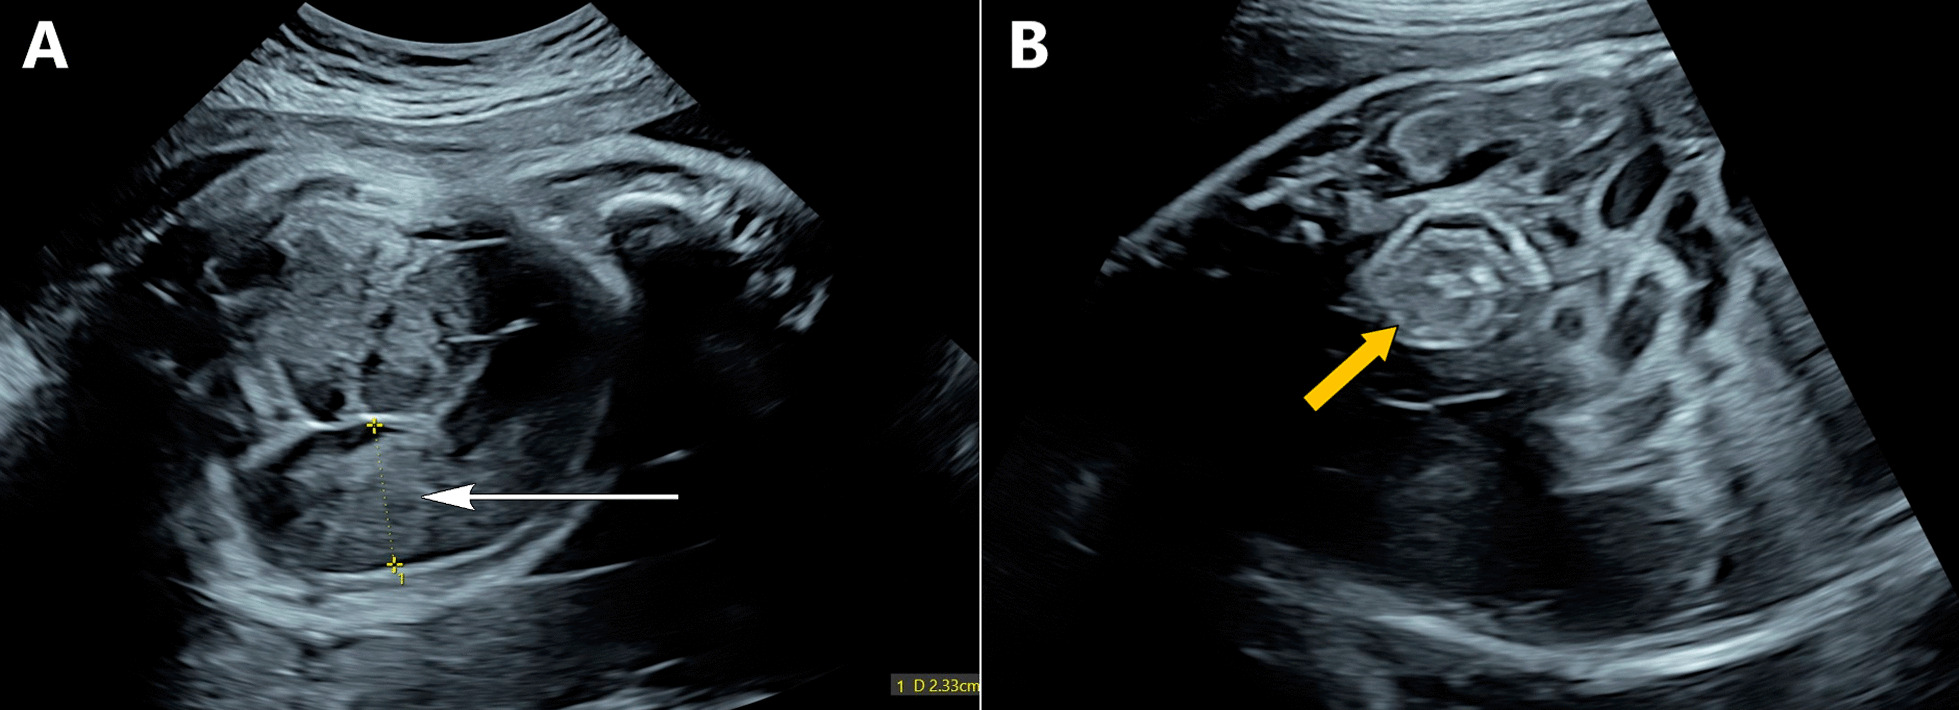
A,B Transverse transabdominal fetal sonography images demonstrating dilated bowel loops (white arrow) up to 2.3 mm (A) and classic “whirlpool sign” of twisted mesentery (yellow arrow) within the fetal abdomen (B)
